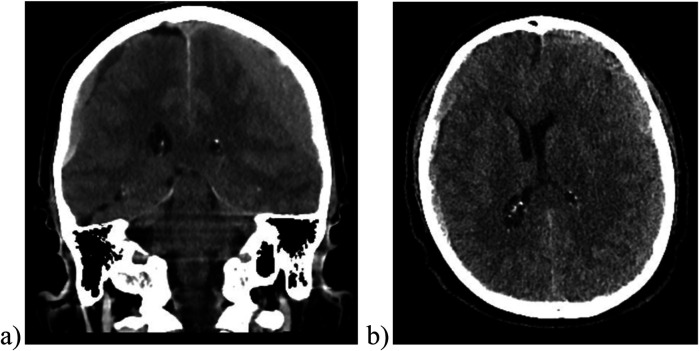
Cranial CT scan images, including coronal (a) and axial (b) views, reveal a bilateral subdural hematoma. Right-sided hematoma measures approximately 0.8 cm, while the left-sided hematoma about 2 cm, resulting in mass effect and midline shift to the right of approximately 0.9 cm.
